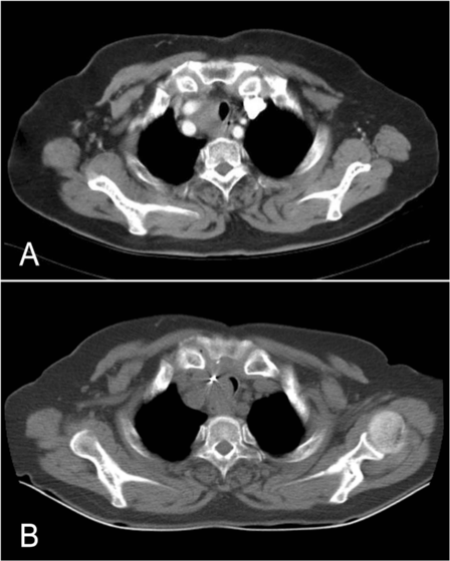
Computed tomography (CT). a Before surgery: enhanced CT scan noted a large substantive mass around the right common carotid artery bifurcation. b Six months after surgery: CT scan showed the trachea narrowed, in the level of the thoracic inlet, next to the silver clips
